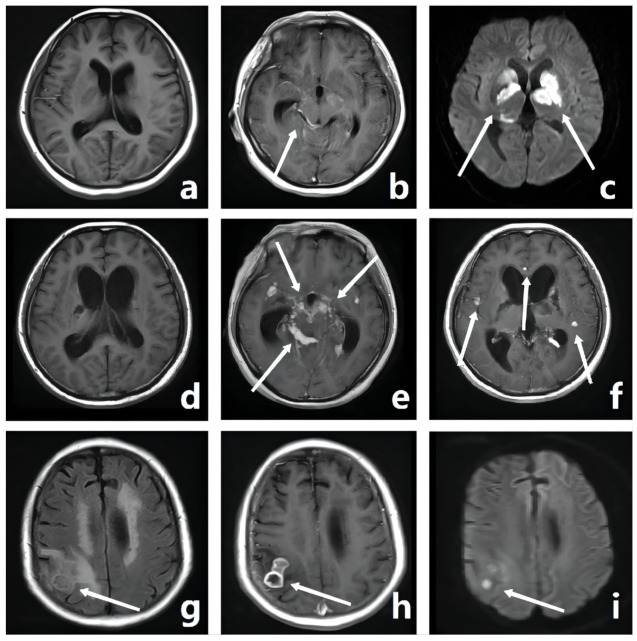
A 26-year-old female. There was exudation in her skull base, and the meninges of the ambient cistern and lateral fissure cistern were enhanced on T1WI ((b), white arrow). She had ACI in the bilateral basal ganglia, which showed hyperintensity on DWI ((c), white arrows). One month later, the patient was reexamined with MRI. She developed moderate hydrocephalus with an Evan’s ratio of 0.35 (d), compared with the initial Evan’s ratio of 0.30 (a). There are countless tuberculomas in the meninges ((e), white arrows) and brain parenchyma ((f), white arrows), showing obvious homogeneous nodular enhancement. Another 70-year-old female. She had several brain abscesses in the right parietal lobe and multiple OCIs in the radiated coronal area ((g), white arrow). The abscess wall showed ring-shaped enhancement ((h), white arrow), and the abscess cavity showed obvious high signal intensity on DWI ((i), white arrow).
